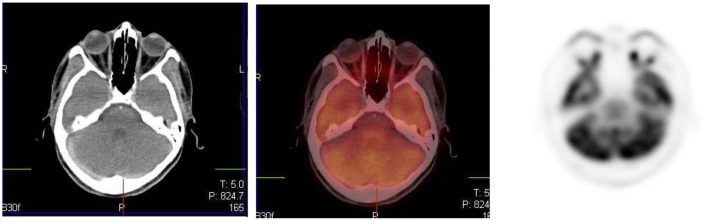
Axial CT, fused PET/CT and PET only images showing physiologic uptake in the medial and lateral ocular muscles.
